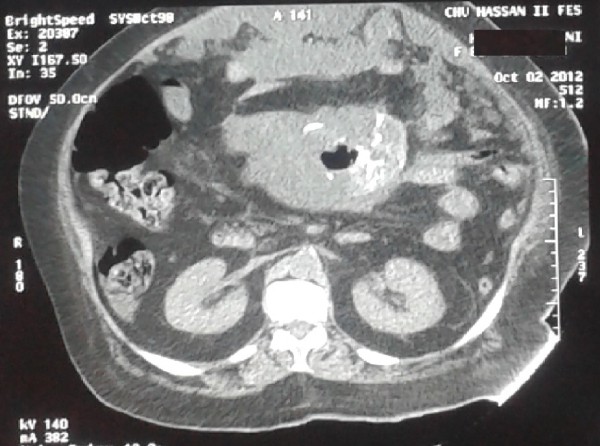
Suspicion of centro tumoral necrosis on CT scan.
